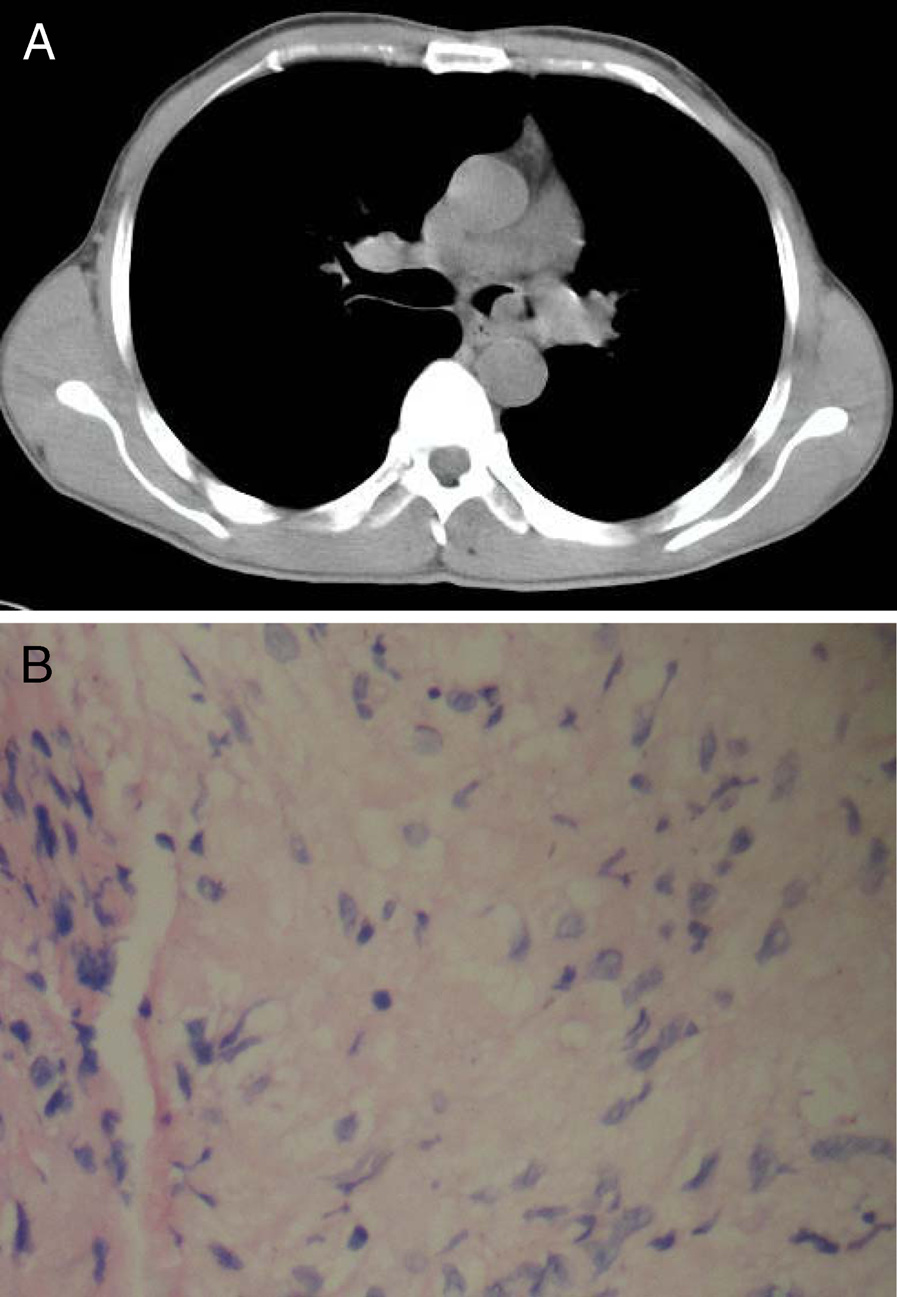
A - Chest CT showing a nodule in left bronchus. B - Pathological section showing interlacing bundles of spindle-shaped cells mixed with collagen. HE × 400.
